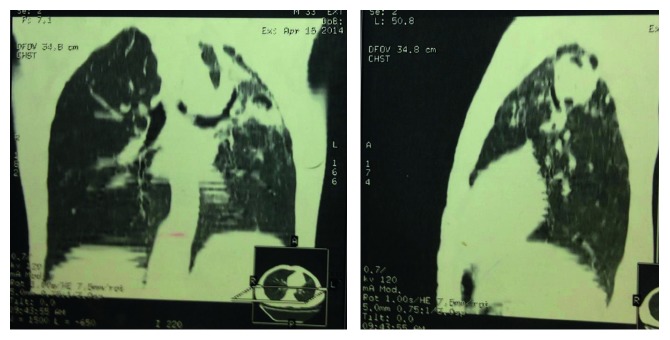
Computed tomography of the chest. A sagittal section showing a left-sided complex lesion of the left upper lobe very close to the aorta.
